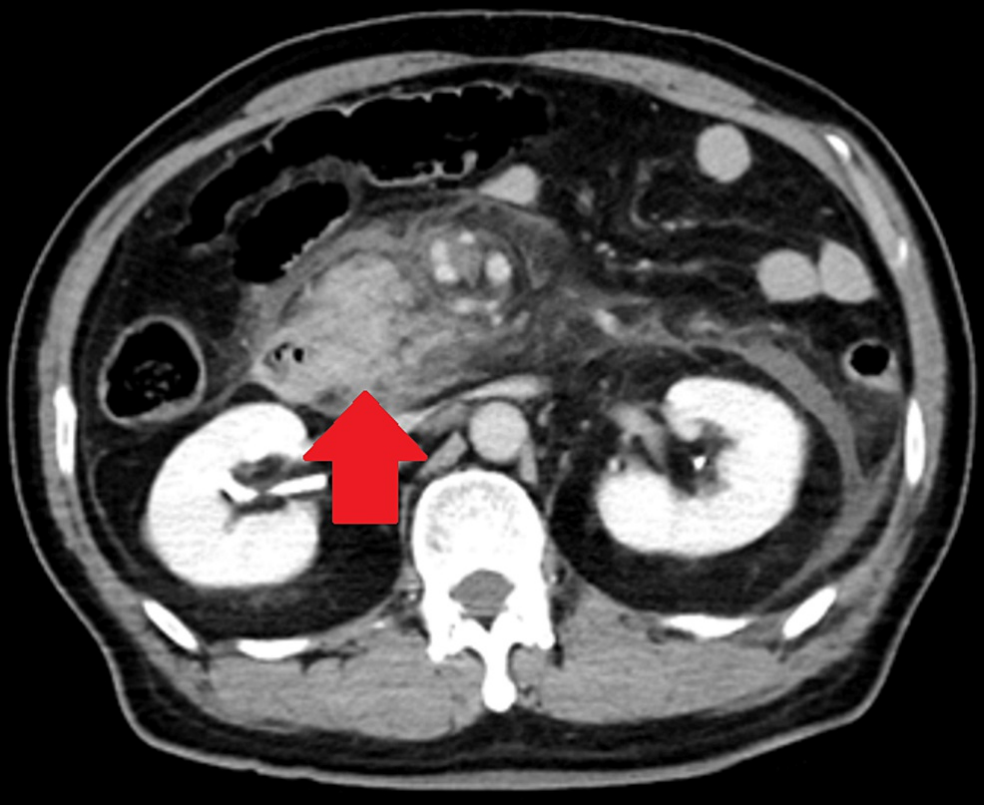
Contrast-enhanced abdominal CT indicating the inflammatory findings in the abdominal cavity (red arrows)
